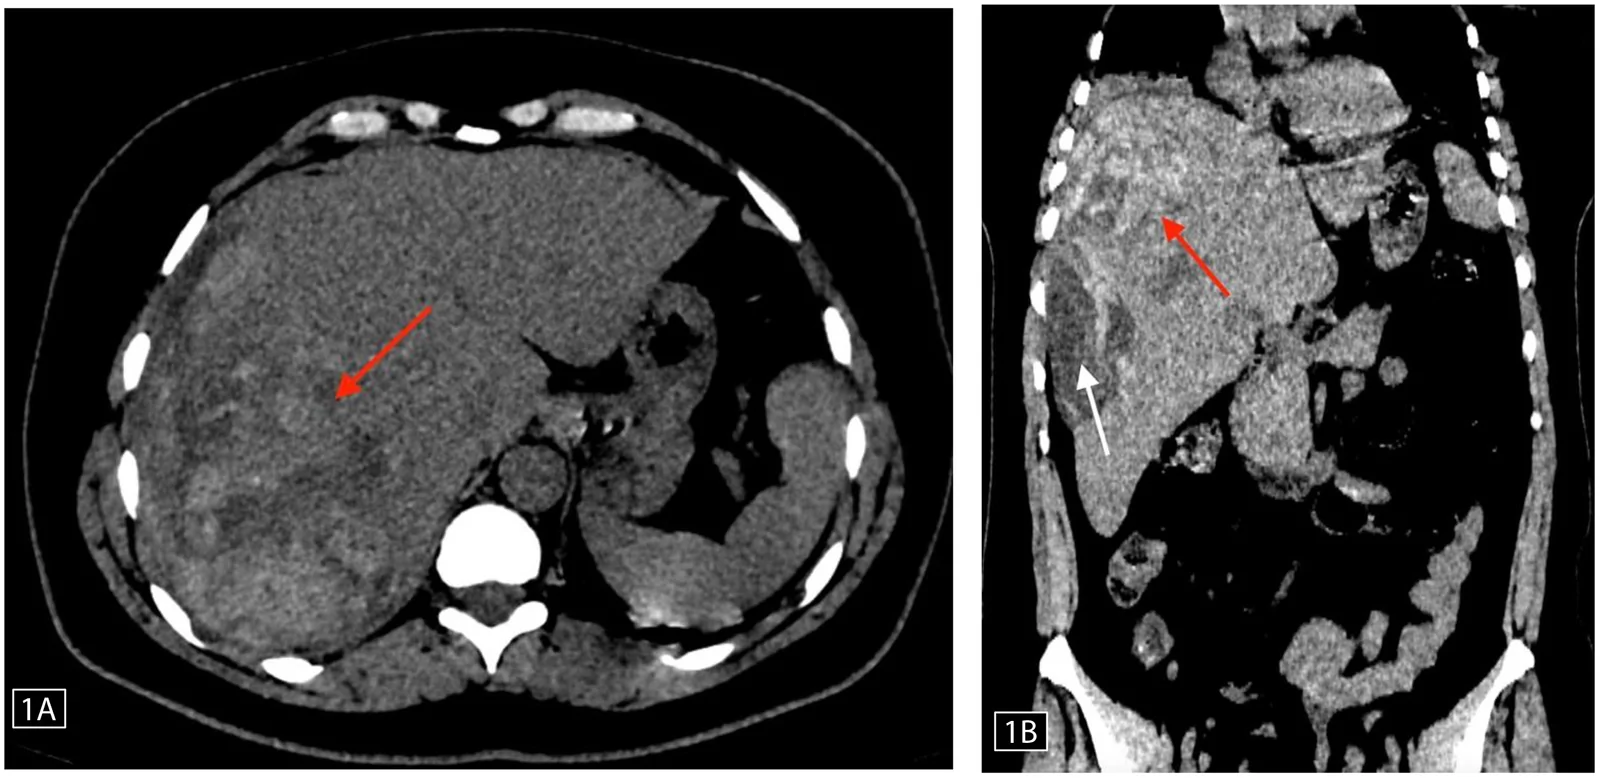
Unenhanced CT appearance of the largest hemorrhagic hepatocellular adenoma. (A and B) Axial and coronal unenhanced CT images showing a 12-cm heterogeneous, spontaneously hyperattenuating lesion involving segments VII and VIII, consistent with extensive intralesional hemorrhage (red arrow). The lesion has a subcapsular component with an associated subcapsular hematoma and causes focal bulging of the hepatic capsule (white arrow).
